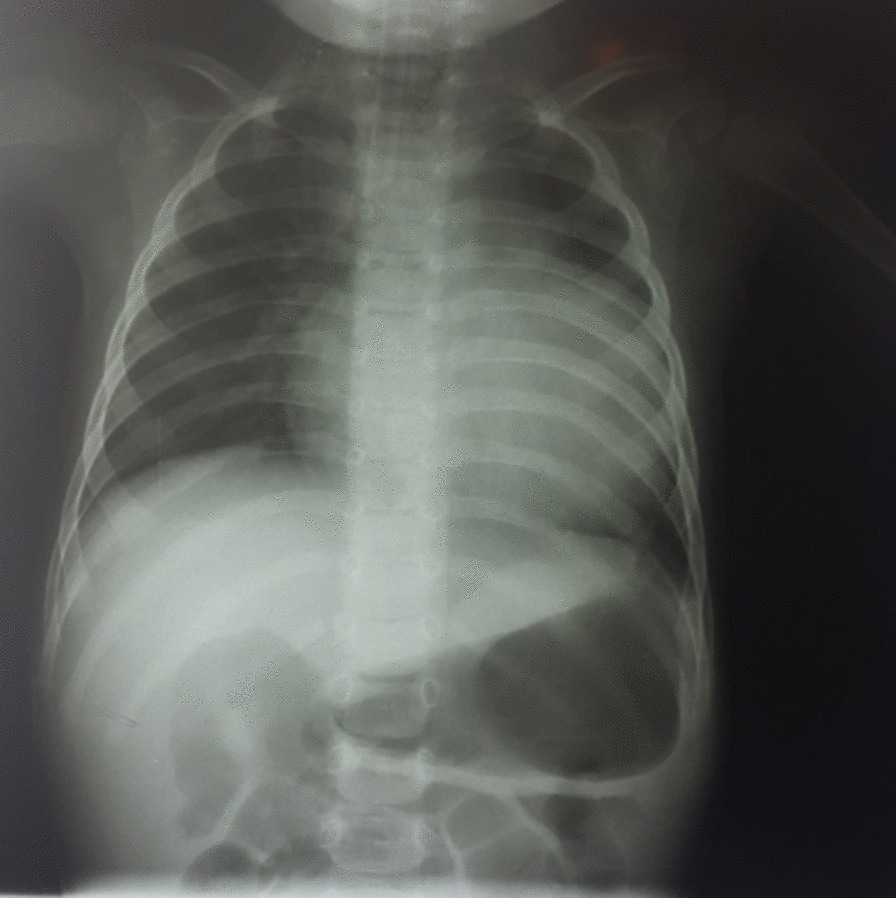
Chest X-ray PA view showing cardiomegaly and hepatomegaly in the patient
